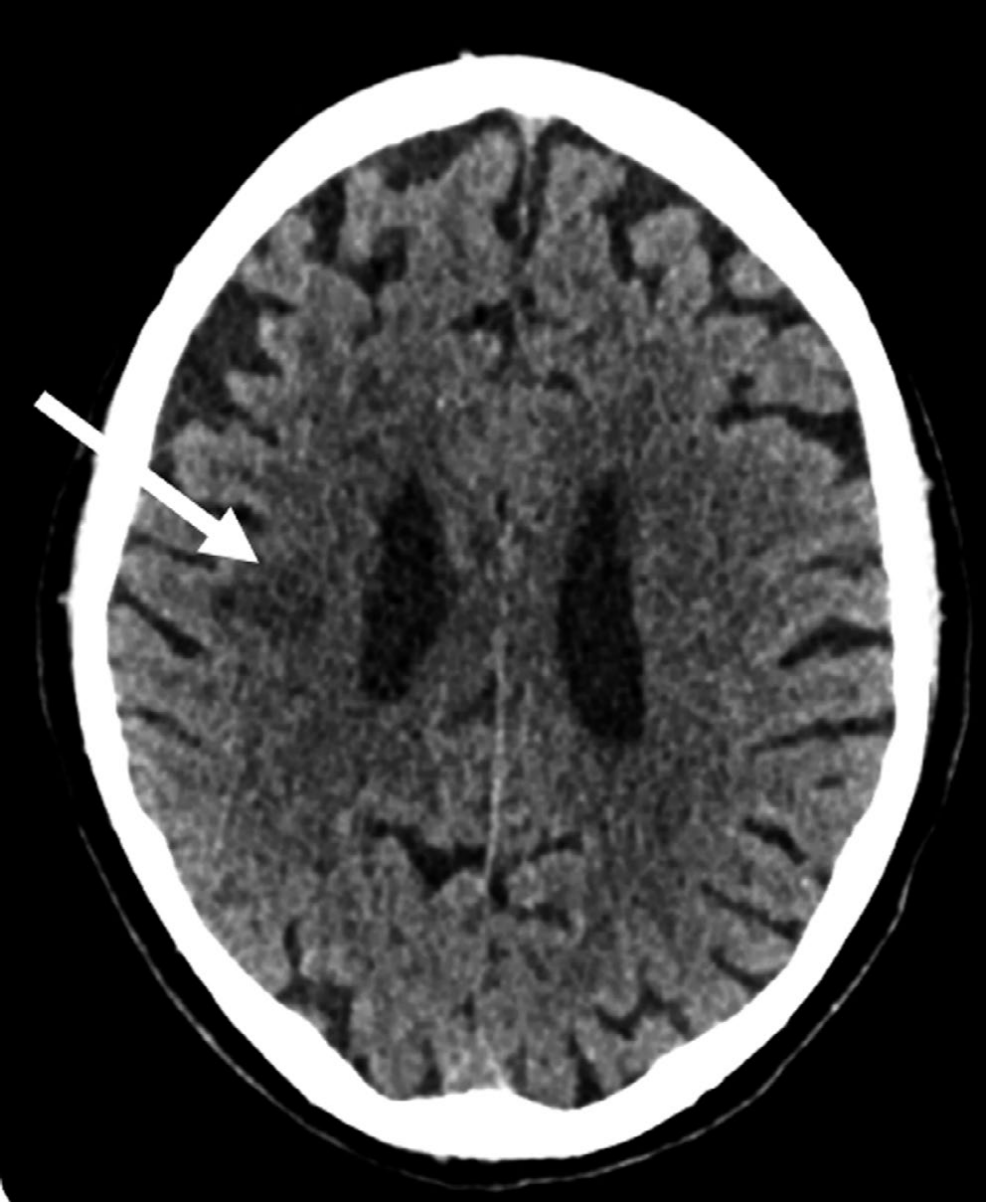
Computerized tomography (CT) scan of the head was performed which showed multiple areas of low attenuation, as shown by the white arrow
